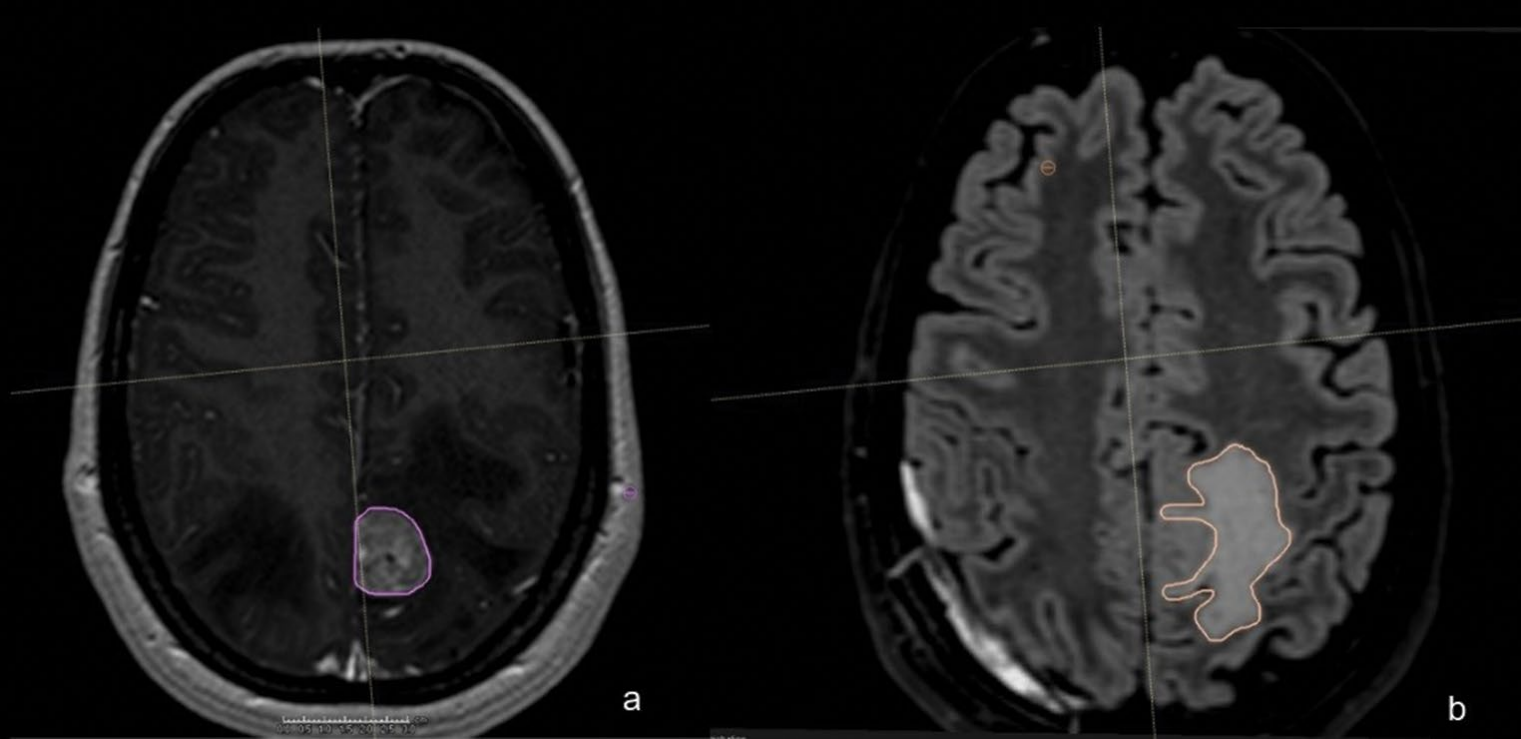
Segmentation of Gross Tumor Volume (GT) on contrast-enhanced T1-weighted images (a) and Perilesional Edema Volume (PEV) on FLAIR-weighted images
